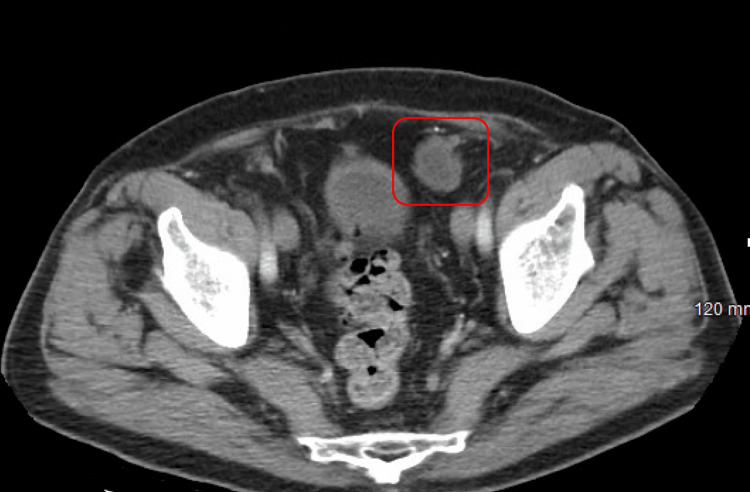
CT of the abdomen and pelvis without contrast (axial view) with recurrent fat-containing left inguinal hernia found in the left anterior pelvis with a 2.7 cm structure consistent with a previous left inguinal hernia repair plug (red box).
